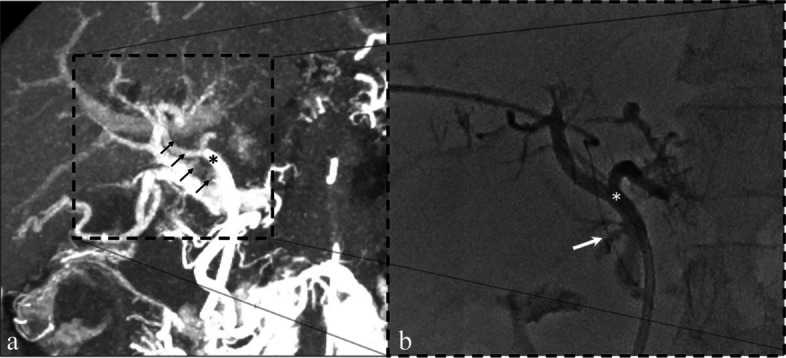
Identification and traversal of a submillimeter cavernomatous collateral. a CT during catheter-directed superior mesenteric arteriography with maximum intensity projection (MIP). The superior mesenteric vein (SMV) is strongly opacified, whereas the intrahepatic portal vein is faintly visualized. An arrow indicates a faint linear structure corresponding to a traversable submillimeter cavernomatous collateral connecting the SMV and an intrahepatic portal branch, which was not appreciated on routine portal venous phase CT. Transhepatic portography obtained through a 1.7-Fr microcatheter (Progreat λ, Terumo Corporation, Tokyo, Japan) advanced from the intrahepatic portal vein into the SMV via the same pathway (arrow), confirming successful intraluminal traversal. Asterisks (*) indicate corresponding distal SMV locations in panels a and b
